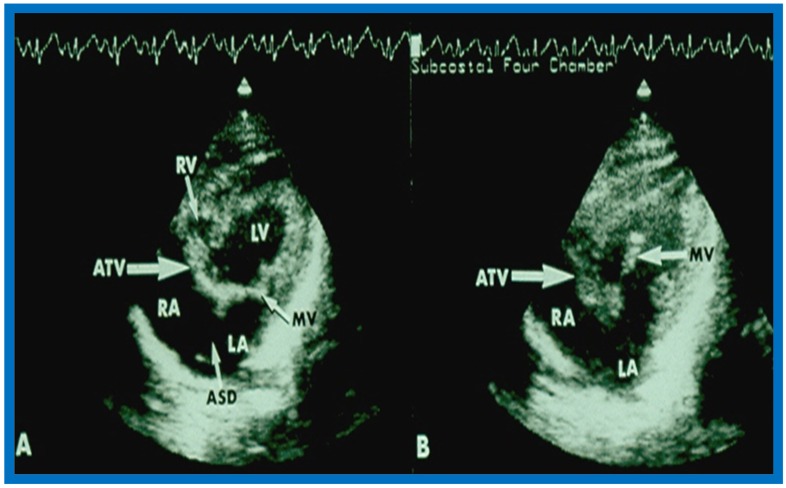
Selected video frames from subcostal four-chamber view of a two-dimensional (2-D) echocardiographic study demonstrating atretic tricuspid valve (ATV) (thick arrow), represented by a dense band of echoes between the right atrium (RA) and hypoplastic right ventricle (RV). In (A), the mitral valve (MV) is closed, while in (B), it is open. Note the improvement from the pictures shown in Figure 14. LA, left atrium; LV, left ventricle. Reproduced from Rao P.S. [35].
